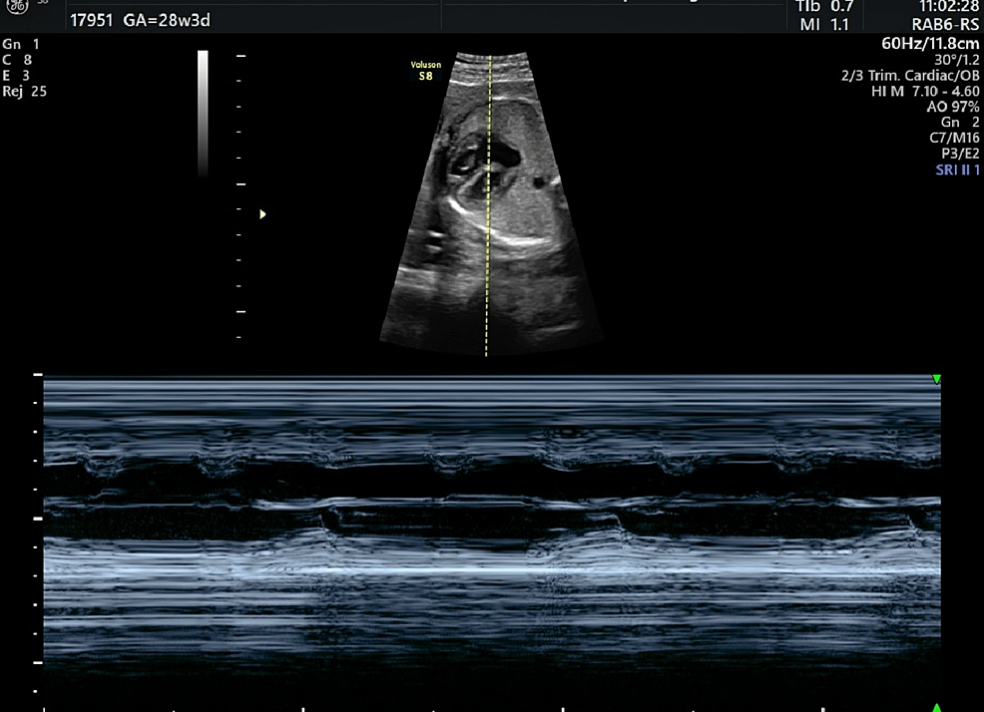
Detailed fetal echocardiography demonstrating the presence of second-degree heart block
